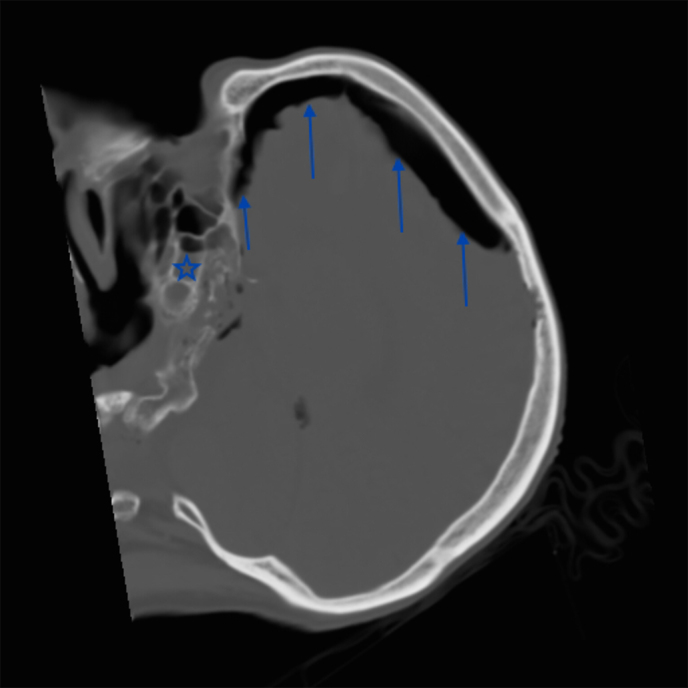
Sagittal CT brain image on bone windows demonstrating a large frontal subdural air collection (arrows) compressing the frontal lobe. The dependent air/fluid level in the sphenoid sinus raises the suspicion of an occult fracture (star).
